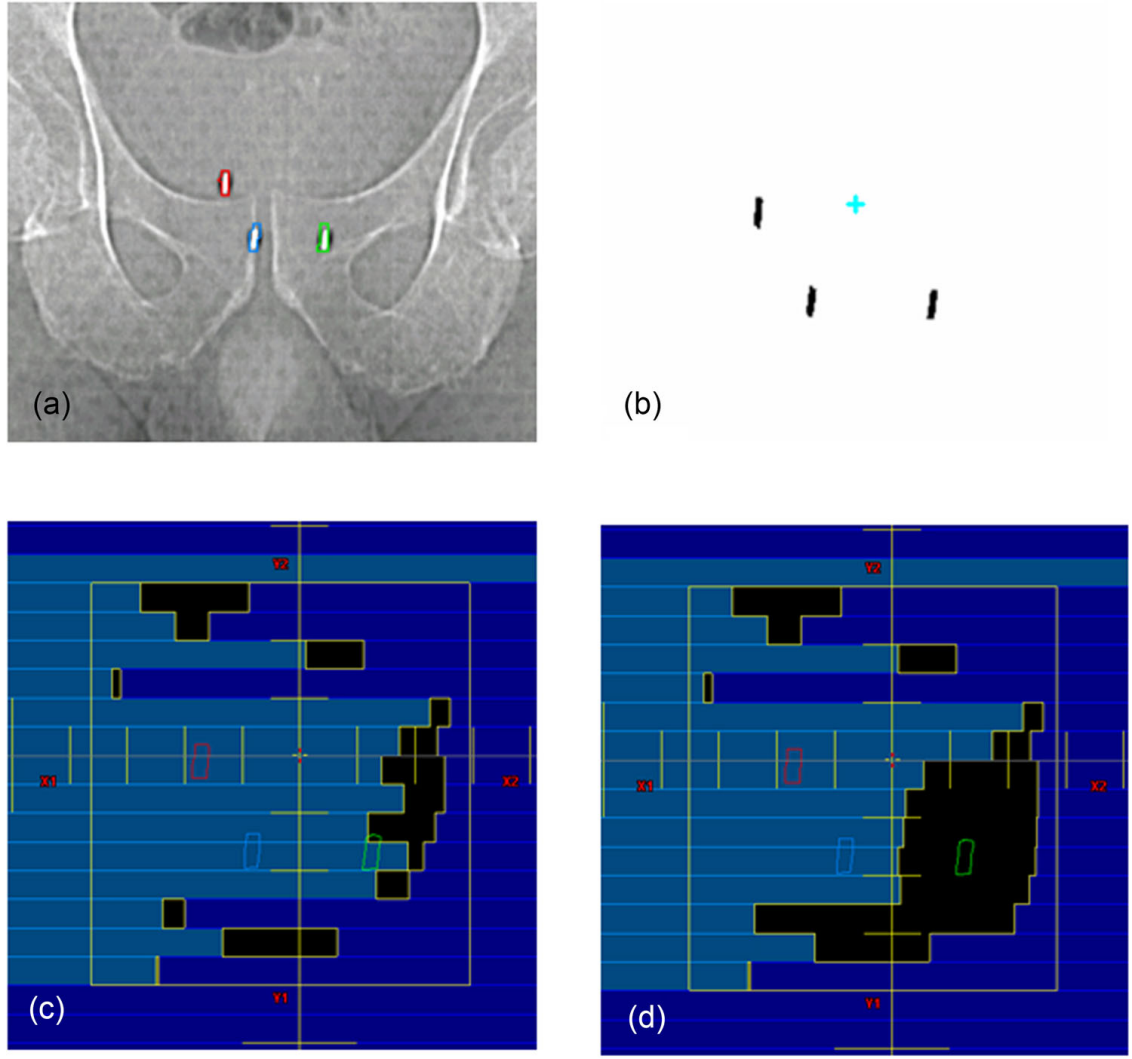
Fiducials prepared for the MV–kV treatment. (a) CT scouts showing three fiducials, (b) fiducial templates, (c) multi‐leaf collimator (MLC) aperture before modification, and (d) MLC aperture after modification
